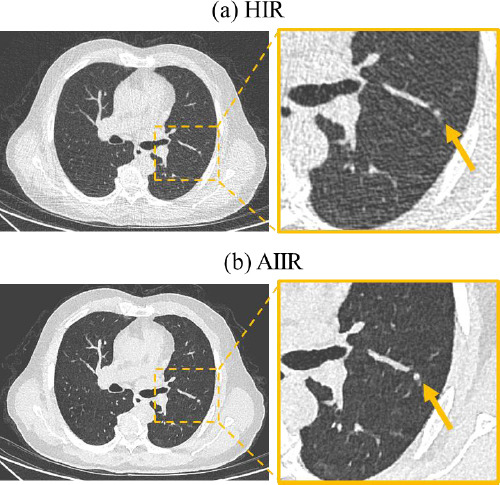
Ultra-low-dose screening chest CT images obtained in a 66-year-old man. Images were acquired at a CTDI_vol_ of 0.22 mGy and reconstructed with HIR (a) and DIR (b). DIR provides a sharper image appearance than HIR. The nodule (yellow arrow) is clearly visualized on the DIR image, whereas on the HIR image, it is obscured by the noise and streaking artifacts.
